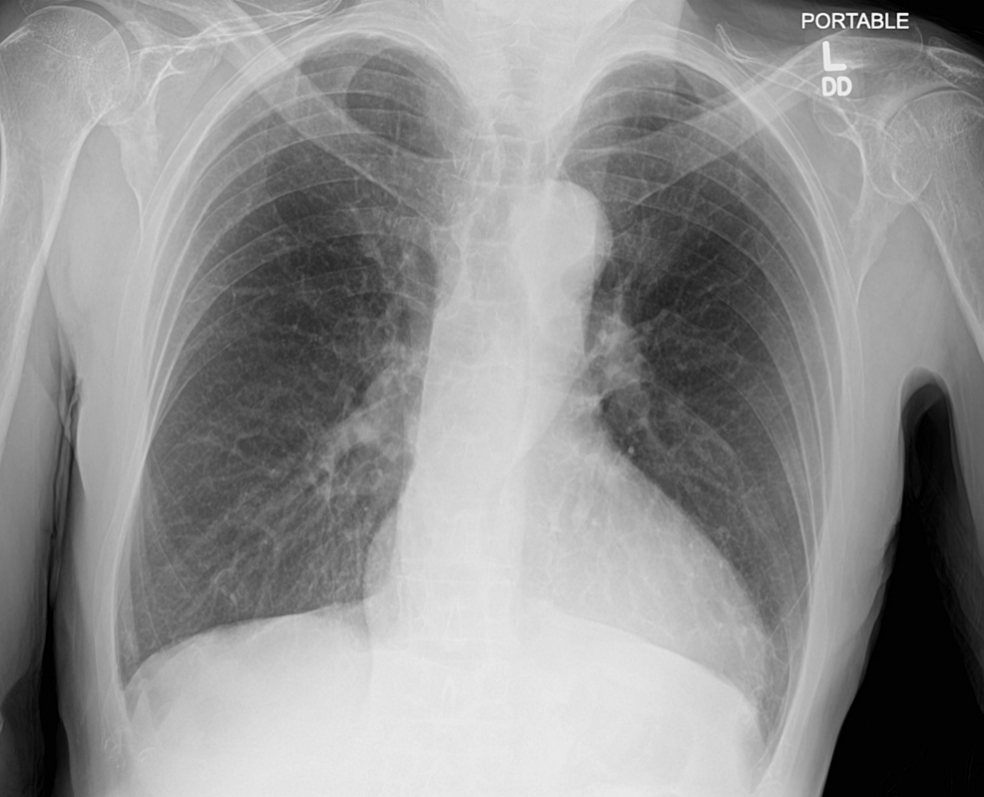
Chest x-ray with no apparent signs of effusion nor consolidation
